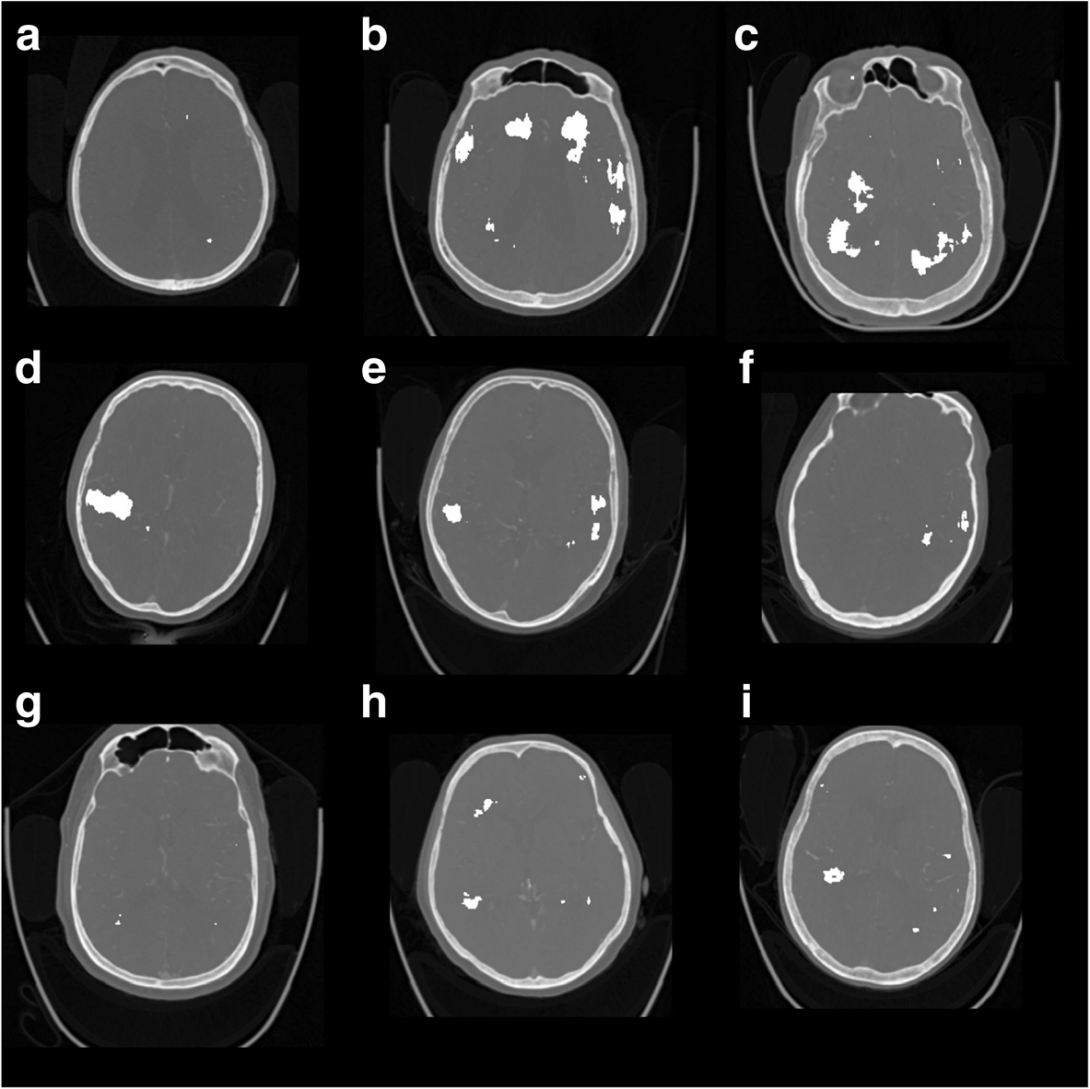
Representative slices of nine (a-i) of the infarct negative test cases. 3D CNN (false positive) output with CTA + cerebral hemispheric comparison + NCCT features are shown in white
